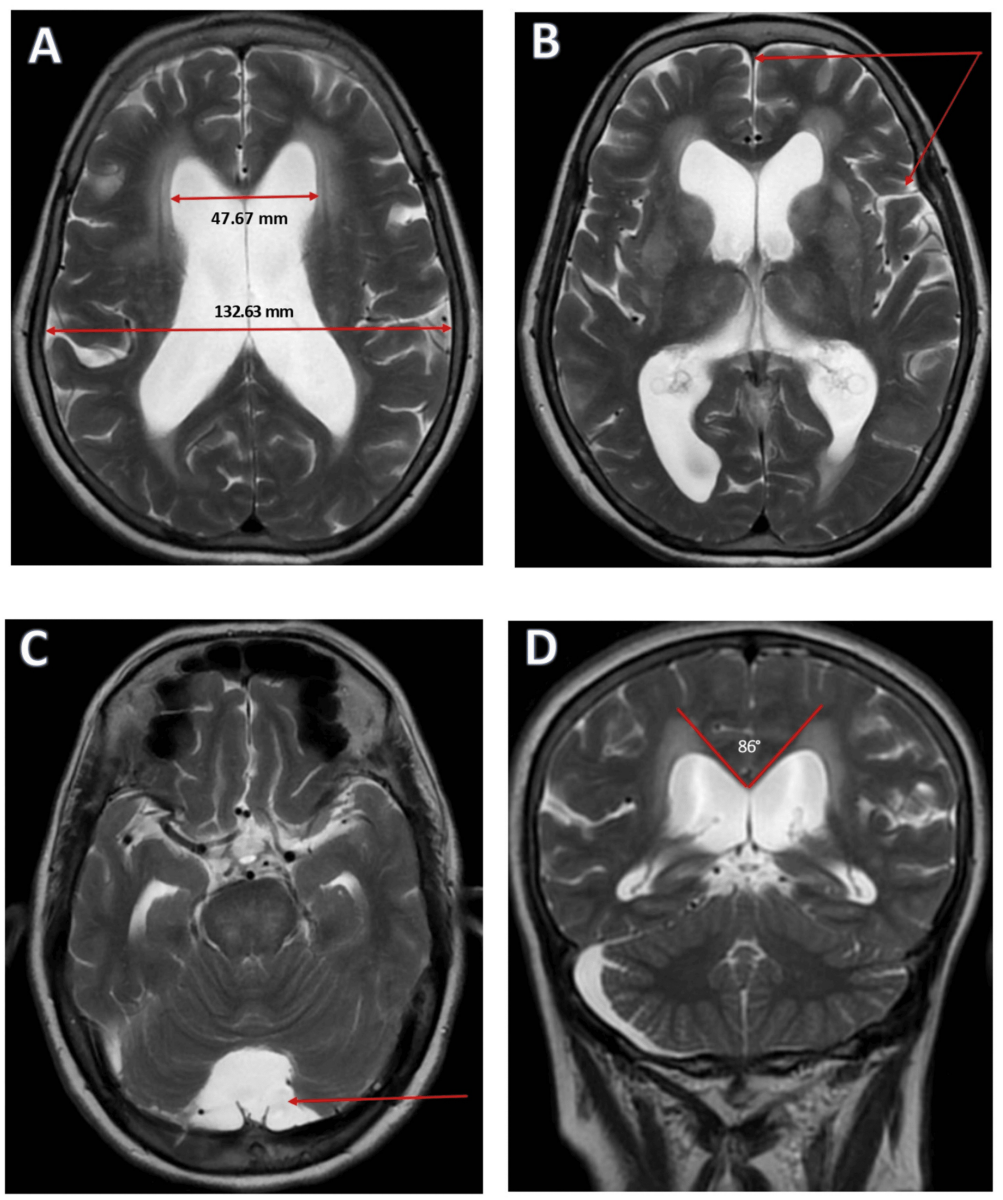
Brain MRI T2-weighted images showing features consistent with iNPH A: Axial T2-weighted image shows marked ventriculomegaly with increased Evans index (ratio of frontal horn width to maximal inner skull diameter, arrows), consistent with hydrocephalus. B: Axial T2 image demonstrating DESH pattern, with narrowing of high-convexity sulci and widened Sylvian fissures, a classic radiological feature of iNPH. C: Axial T2 image at the level of the posterior fossa reveals an enlarged fourth ventricle consistent with communicating hydrocephalus. D: Coronal T2-weighted MRI showing a narrow callosal angle (<90°), which is characteristic of iNPH. MRI: magnetic resonance imaging, iNPH: idiopathic normal pressure hydrocephalus, DESH: disproportionately enlarged subarachnoid space hydrocephalus
